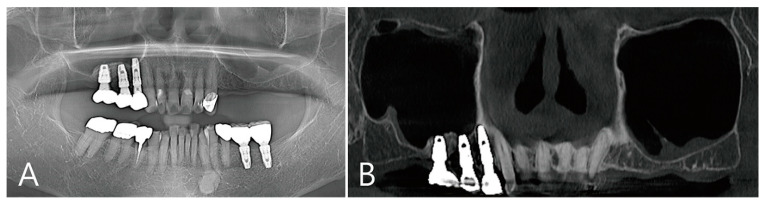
Case 4. Preoperative panoramic radiography and CBCT scan were performed: (A) the left maxillary sinus was severely pneumatized and had minimal residual bone height; (B) in the maxillary sinus, membrane thickening was confined to the sinus floor and no sinus pathology was observed in the remaining areas.
